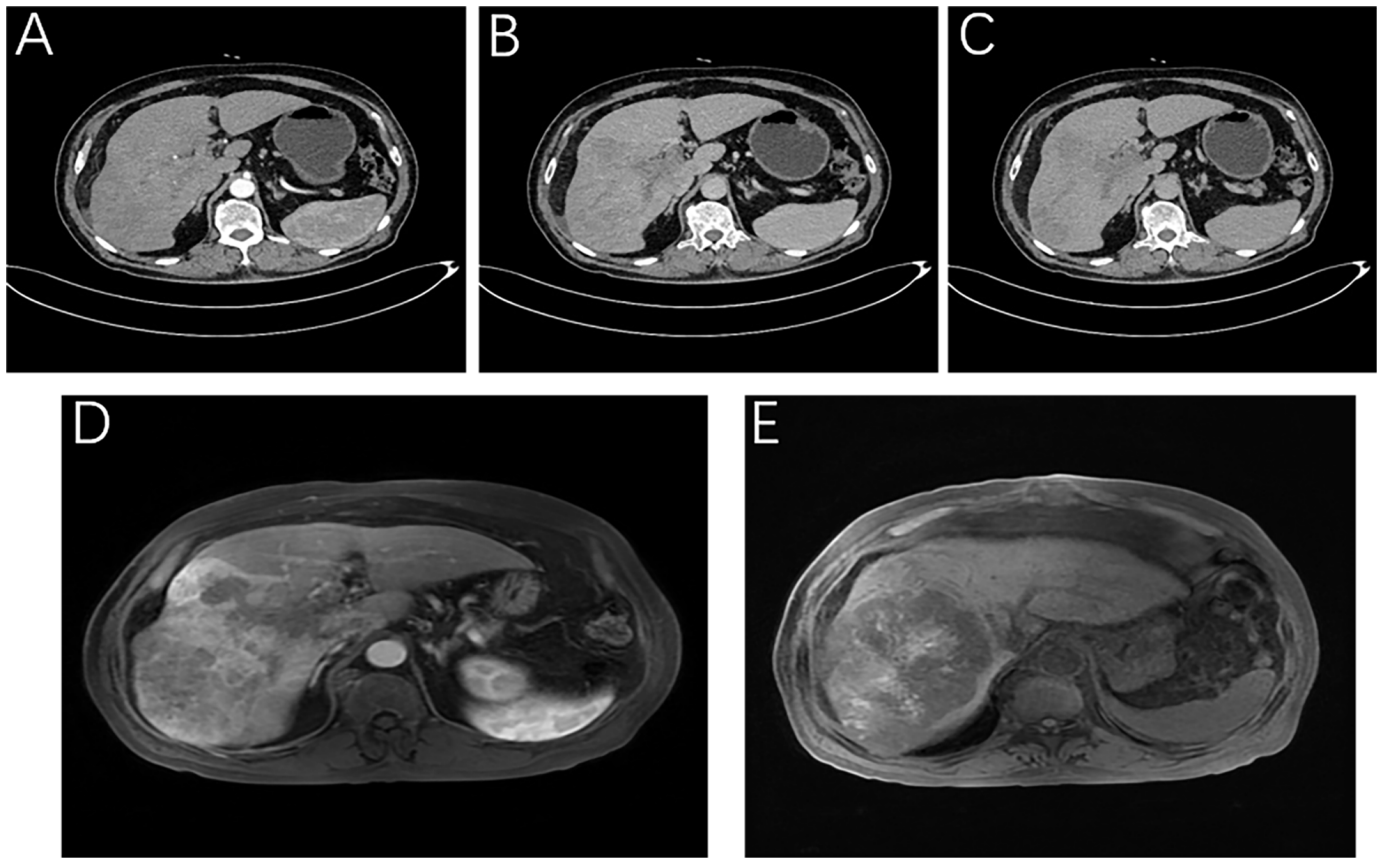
Imaging of the patient at the time of initial diagnosis. Liver-specific MRI: (A) Arterial phase, (B) Portal vein stage (blood vessel), (C) Grace; Enhanced abdominal CT: (D) Portal stage: portal vein thrombosis, (E) T1WI intratumor hemorrhage.
